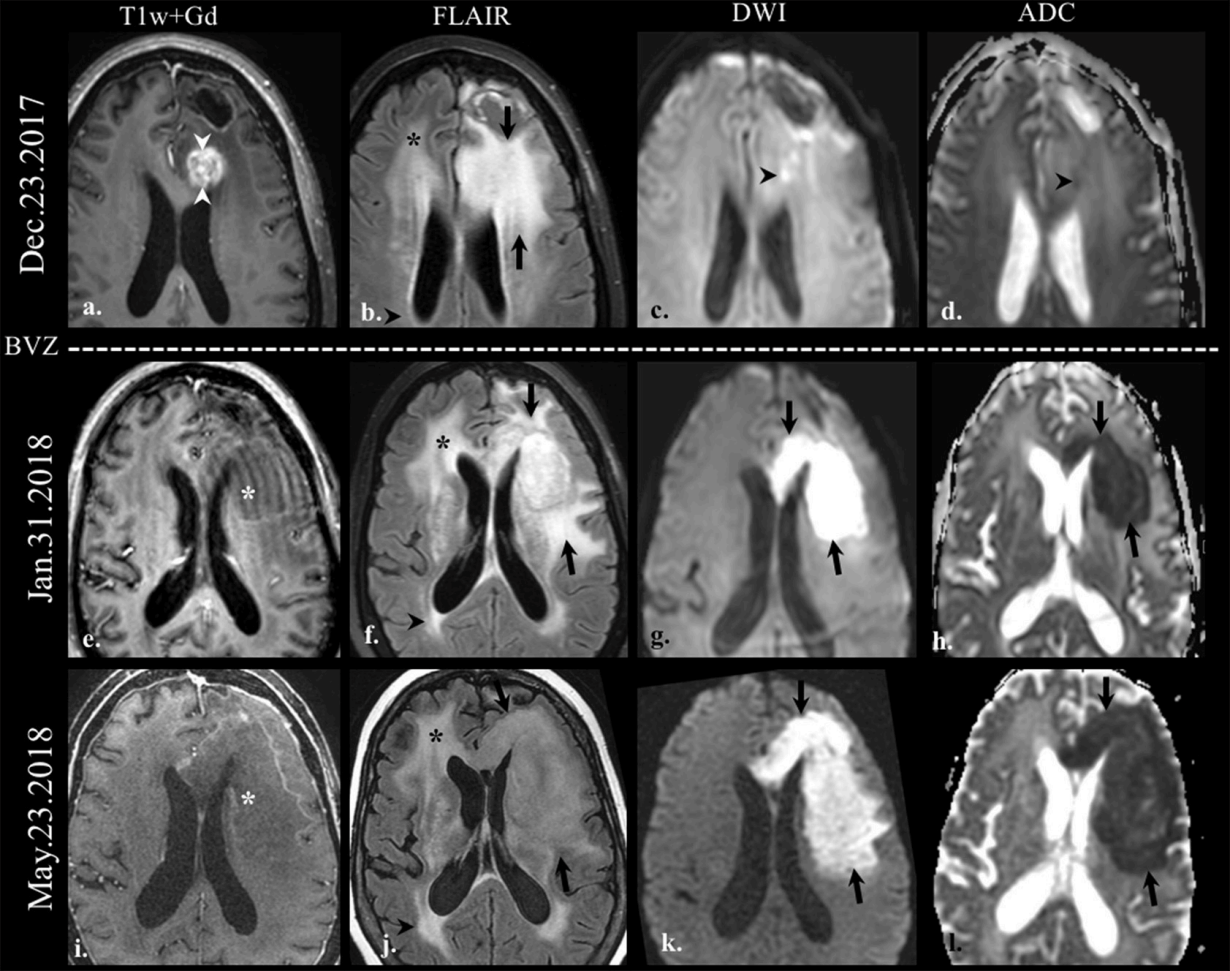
Diffusion-weighted imaging (DWI) in pseudoresponse. Brain MRI of a old patient with the history of GBM (IDH wild-type, MGMT status unknown) on the first recurrence. Axial gadolinium-enhanced T1w (a,e,i), FLAIR (b,f,j), DWI (c,g,k), and apparent diffusion coefficient map (d,h,l) images are shown. The baseline scan (top row) shows FLAIR hyperintensity (arrows on b) involving the left frontal lobe adjacent to the posterior margin of the surgical cavity, along with an area of enhancement (arrowheads on a). There is a small focus of DWI hyperintensity (arrowhead on c) with its corresponding hypointensity on the ADC map (arrowhead on d) indicative of restricted diffusion. On follow-up scans after bevacizumab treatment (BEV) the enhancement disappeared (*on e,i); however, the FLAIR hyperintensity increased in size on the left cerebral hemisphere (arrows on b,f,j). Note the striking increase in the size of the area of restricted diffusion on follow-up scans (arrows on g,k, h,l) despite the absence of enhancement; this finding is indicative of the hypercellular nature of the tumor. There is non-specific progressive FLAIR hyperintensity on the right frontal lobe (*on b,f,j), and on the right periatrial region (arrowheads on b,f,j), without associated enhancement or restricted diffusion, likely treatment-related.
